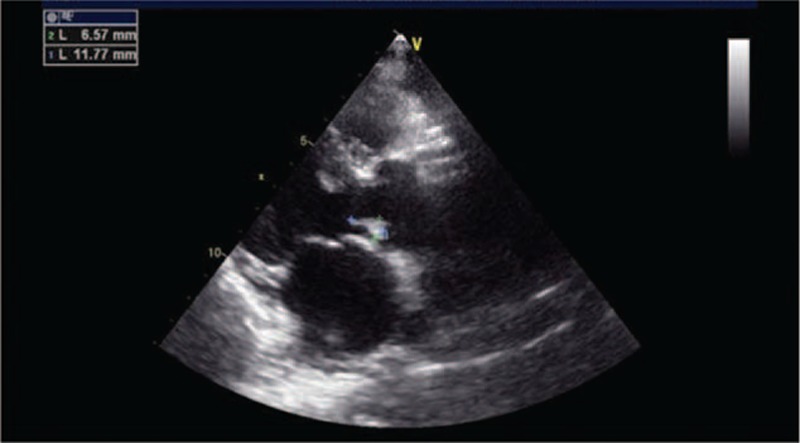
The echocardiogram showed a strong echo of 1.17∗0.66 cm at the valvula coronaria sinistra valvae aortae.
